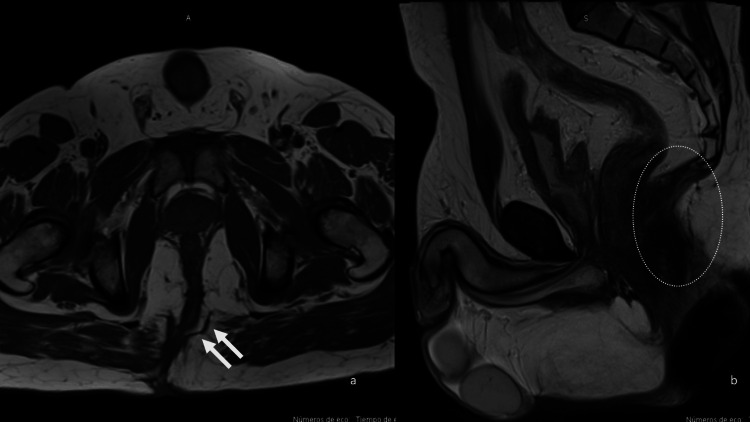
Pelvic MRI showing a recurrent high posterior transsphincteric fistula (June 2024) Axial T2-weighted image demonstrating a hyperintense tract traversing the external and internal anal sphincters, consistent with a high posterior transsphincteric fistula; see arrows (a). Sagittal view revealing extension of the fistulous tract toward the puborectalis muscle, with associated sphincter complex discontinuity and perianal fat infiltration, see circle (b). MRI, magnetic resonance imaging.
